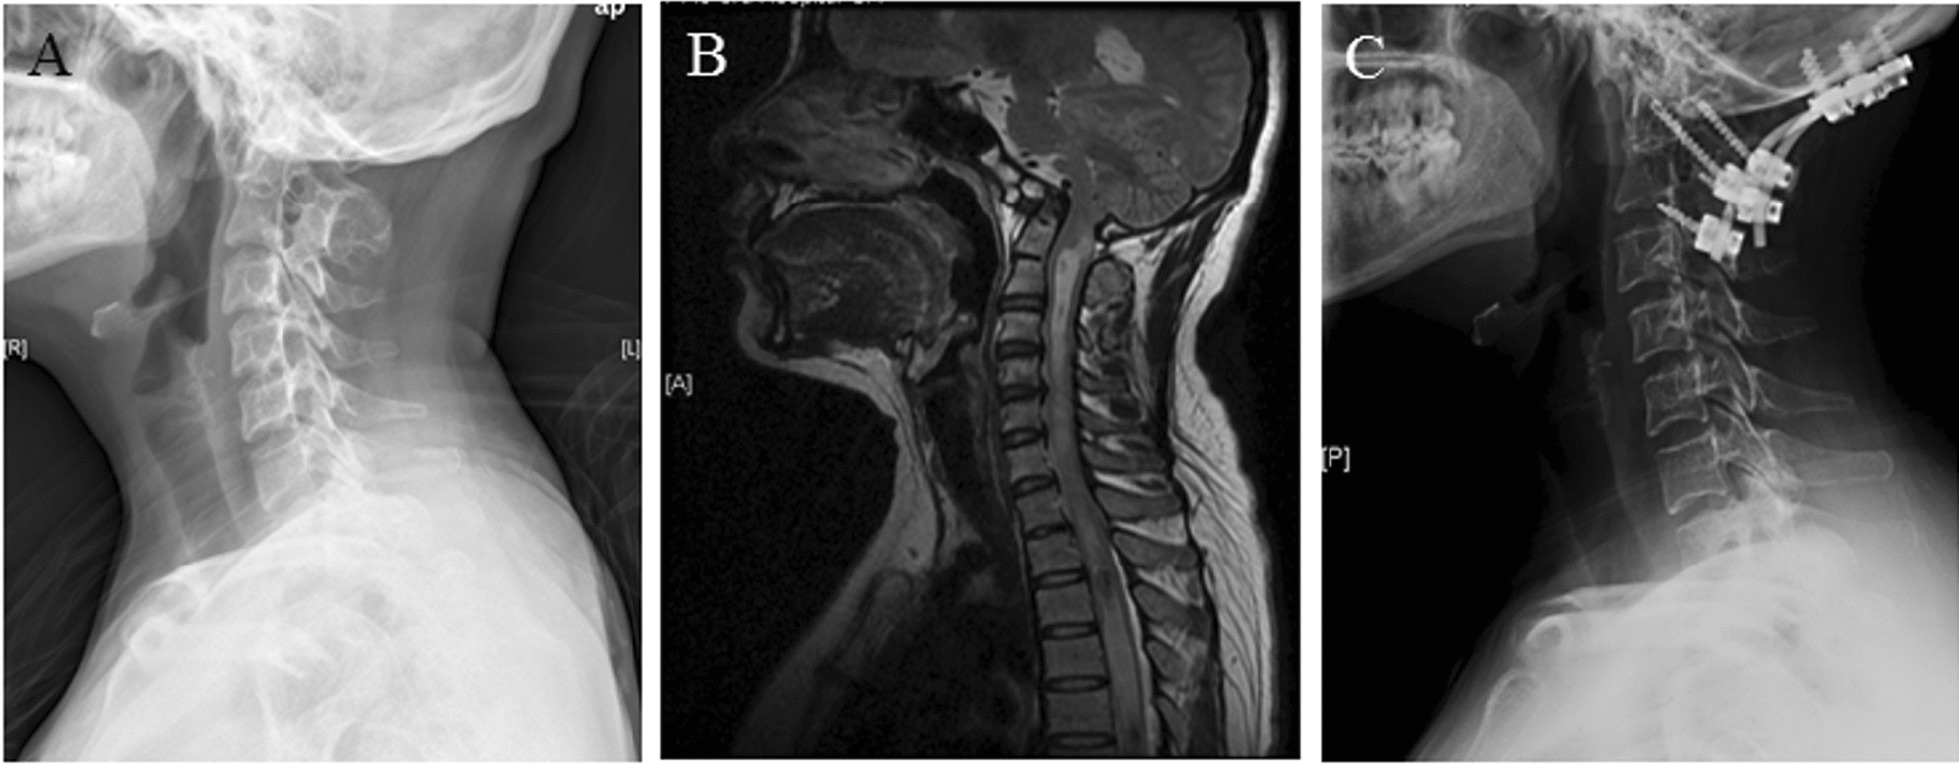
A Pre-operative lateral X-ray of the cervical spine demonstrating the classic “sandwich fusion” of both C0-1 and C2-3 and atlantoaxial dislocation. B Pre-operative sagittal reconstruction of MRI of the cervical spine demonstrating basilar invagination as well as anterior compression of the medulla by the cranially migrated odontoid tip. There was also significant syringomyelia. C Post-operative lateral X-ray of the cervical spine at the one-year follow-up demonstrating maintenance of reduction of atlantoaxial dislocation by occipito-cervical fixation consisting of six occipital screws, two C2 pedicle screws, and two C3 lateral mass screws
